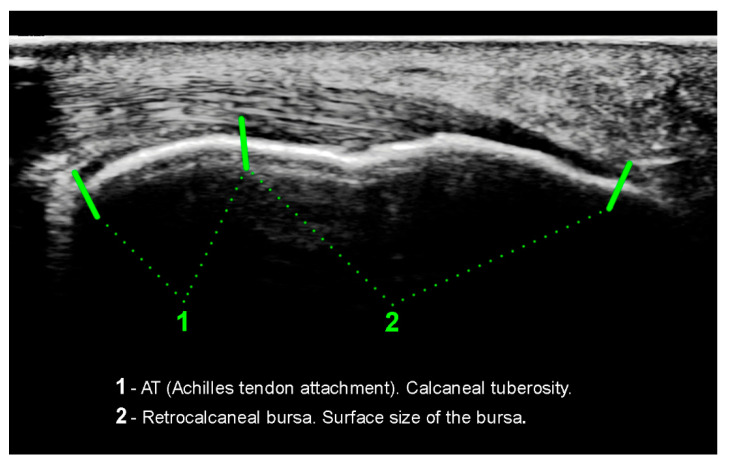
Ultrasonographic image presenting the bursa size measurement.
